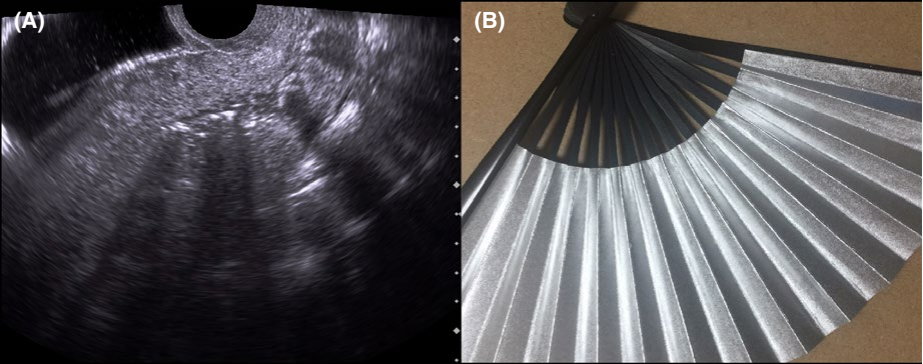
A, Ultrasonography on postpartum day 4. Acoustic shadows originating from the low intrauterine cavity were observed. B, An open fan
